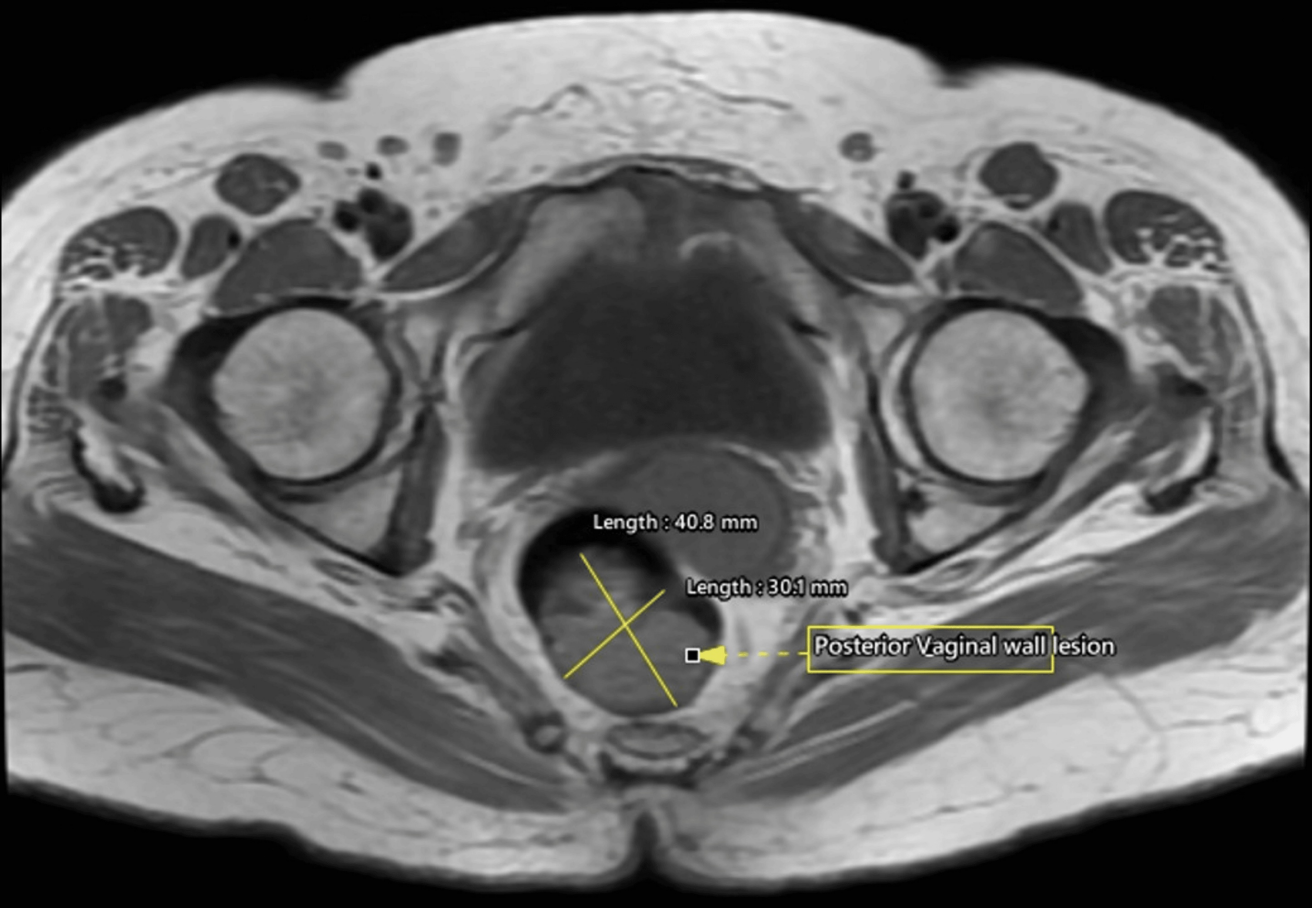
Magnetic resonance imaging (MRI) axial T1 images of the pelvis at the level of the vagina demonstrating a well-defined iso- to hyperintense lesion along the posterior wall of the vagina (yellow arrow)
